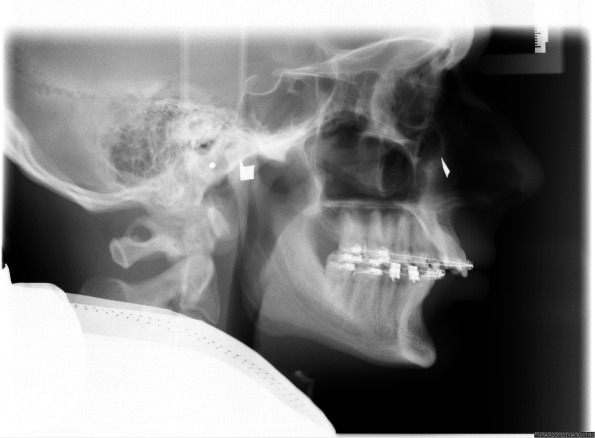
Clinical approach to the determination of the axis orbital plane. The individual hinge axis and the right palpable infraorbital rim were indicated with metal markers on the patient’s skin before a cephalometric radiograph was obtained. Square and triangular markers were used to distinguish between left and right side
